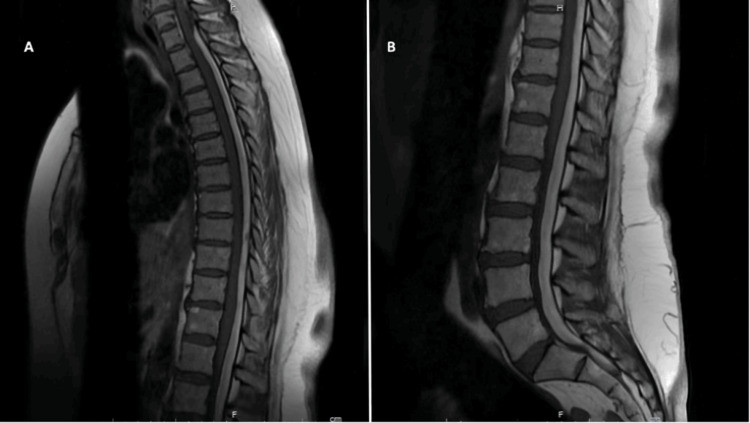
MRI T1 weighted sagittal view of the thoracic (A) and lumbar (B) spine showing acute SSDH MRI: magnetic resonance imaging, SSDH: spinal subdural hematoma
